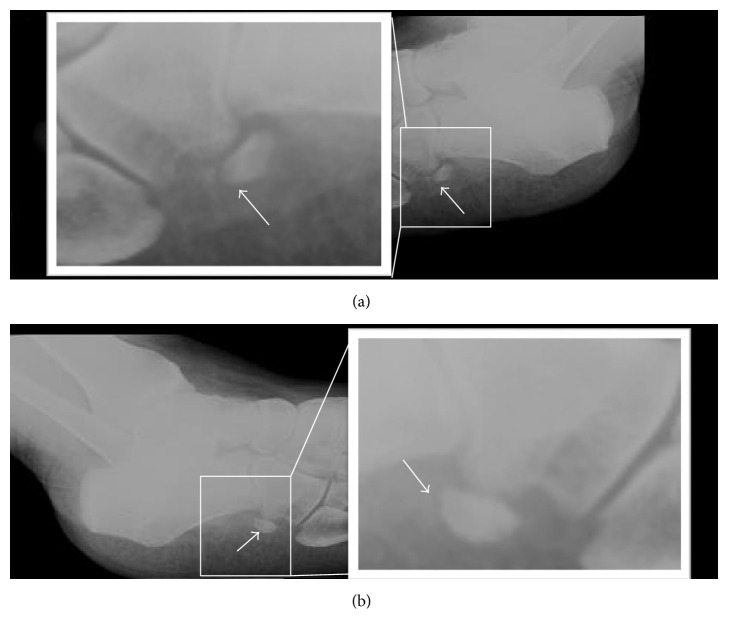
60-year-old female plain film of the feet in an oblique view. (a) Right foot: complaint side, showing an irregular and fragmented os peroneum with heterogeneous density (arrow). (b) Left foot: comparative contralateral side, showing a regular and complete os peroneum with regular contours and homogeneous density (arrow).
